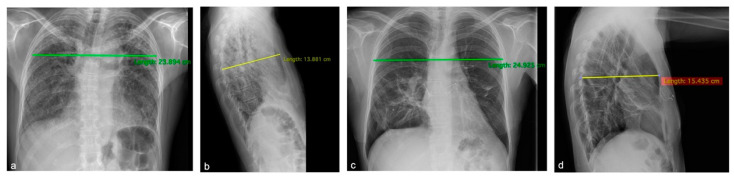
Patient Nº3. Chest X-rays pre- (a,b) and 18 months post- (c,d) lung transplantation show the modifications of the thoracic cage diameters with a reversion of the flat chest.
